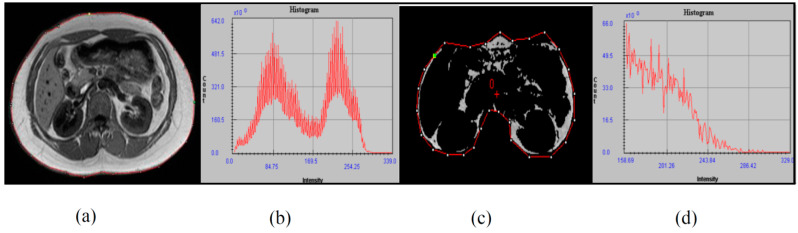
The image was analyzed using Medical Image Processing, Analysis, and Visualization-software package. (a) T1 weighted DICOM MRI image at the L3₋L4 level, (b) total abdominal tissue-related histogram, (c) segmented image for visceral adipose tissue, and (d) related histogram.
